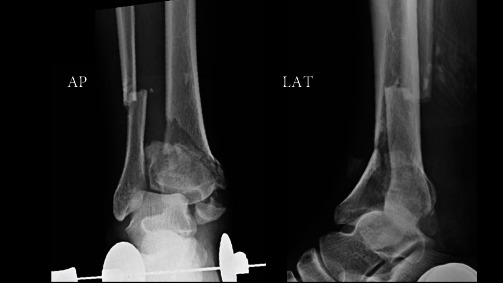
X-rays at the time of injury (after calcaneal traction) show the tibial pilon fractures (AO/OTA 43c3.3, Ruedi–Allogoer: Type III).
